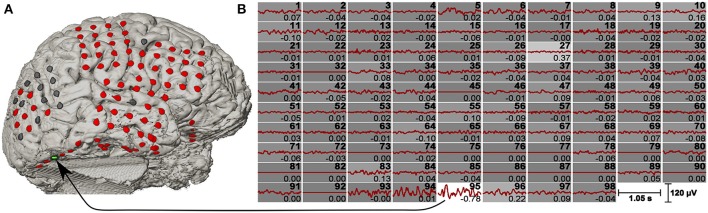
c-VEP templates and spatial filter. (A) Co-registered brain model of a pre-surgical T1-weighted MRI and a post-implantation CT scan. The channels are highlighted in different colors (bad channels are gray, normal channels are red, and the maximum weighted channel of the CCA is green). (B) Signals of all 98 channels were averaged over 200 stimulation sequences. Channel numbers are shown on the top right corner of each plot, while the spatial filter coefficients are shown on the bottom right corner. Beside the numeric value, the spatial filter coefficients are also represented by the background intensity of each channel (the highest weight is plotted on white background). Bad channels were set to zero.
